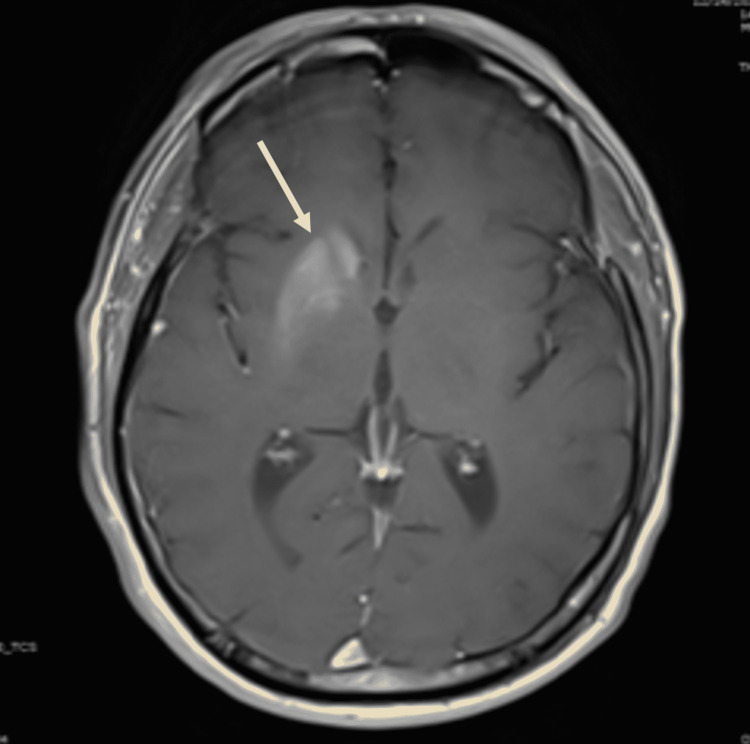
T1-weighted MRI of the brain showing hyperintensity in the right putamen and caudate nucleus (arrow).
